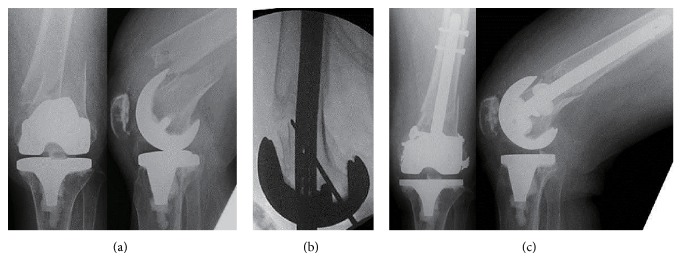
(a) Radiographs of the left displaced periprosthetic supracondylar fracture. (b) A 2.4 mm block pin inserted on the lateral side of the distal fragment. (c) The nail inserted with excellent repositioning of the fracture site.
